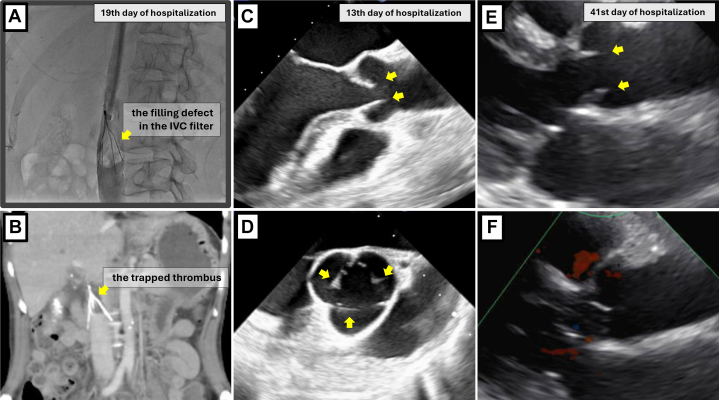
Imaging After Tumor Resection (A and B) Attempted removal of the inferior vena cava (IVC) filter using a catheter was unsuccessful because of a trapped thrombus, seen as a filling defect. (C and D) Regression of aortic valve vegetations (arrows) observed intraoperatively by TEE on the 13th day of hospitalization. (E and F) Complete resolution of all 3 aortic valve vegetations (arrows) by TTE at hospital discharge on the 41st day of hospitalization. TEE = transesophageal echocardiography; TTE = transthoracic echocardiography.
